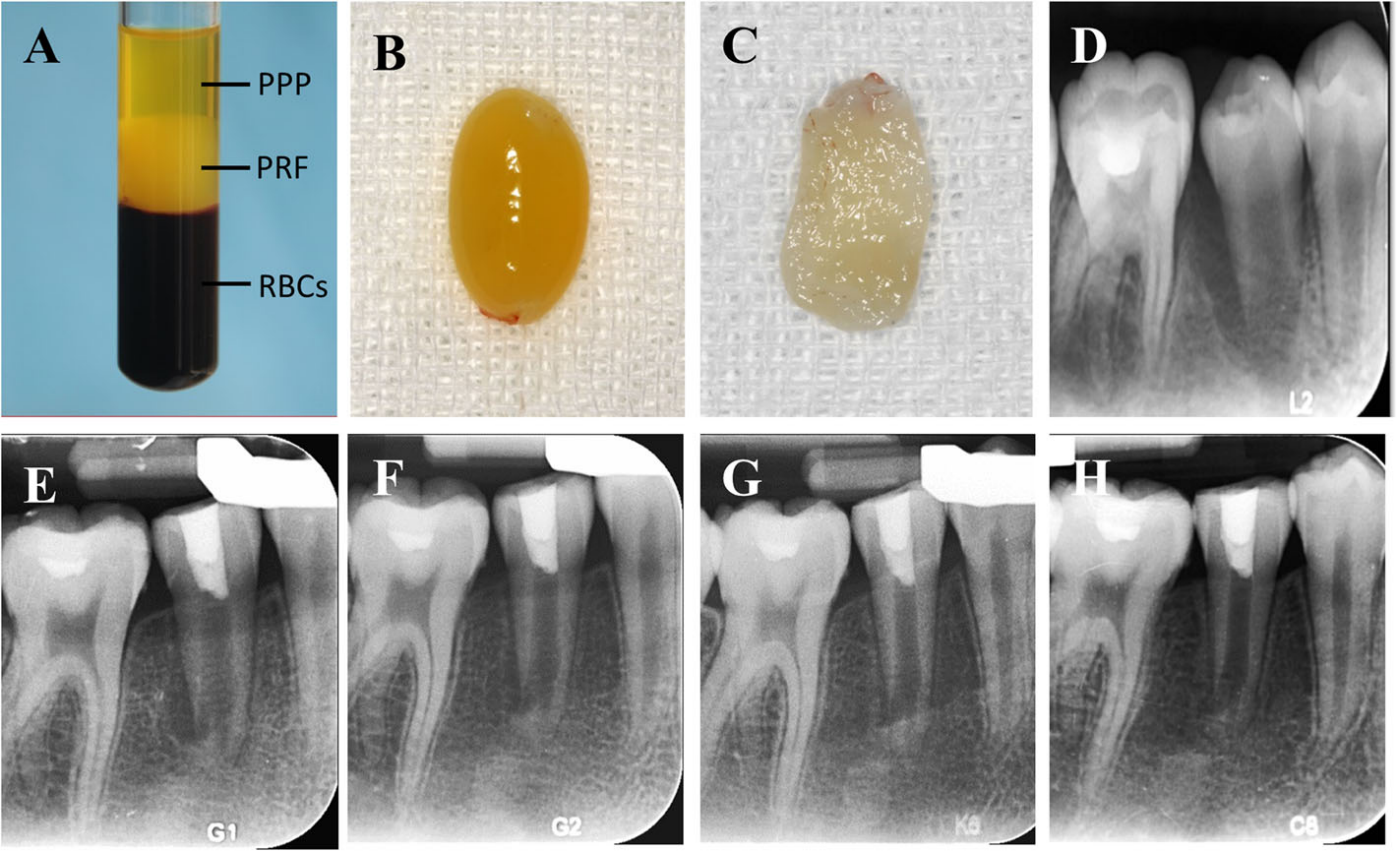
a Peripheral blood after centrifugation: red blood cells at the bottom, PRF in the middle, and platelet-poor plasma at the top. b PRF clot. c PRF membrane. d An periapical radiograph of #45 with apical periodontitis in a 12-year-old girl. The case was treated by RET+PRF in #45. e Three-month follow-up periapical radiograph of tooth #45. f Six-month follow-up radiograph. g Nine-month follow-up radiograph. h Twelve-month follow-up radiograph showing complete periapical radiolucency resolution, root apex closure, root elongation and root canal wall thickening
